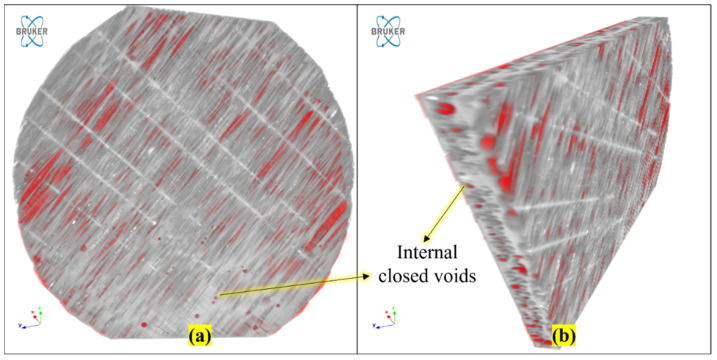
CT-scanned image of Cyperus pangorei rottb fiber panel with internal voids.
